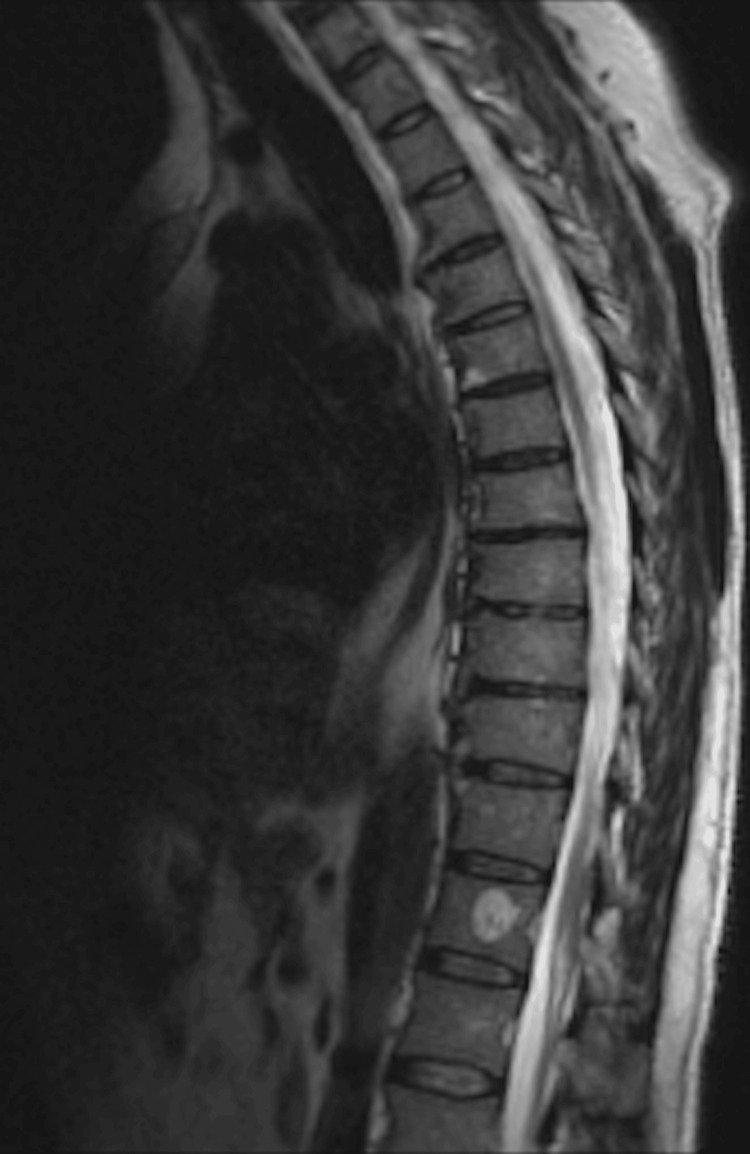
MRI of the thoracic and lumbar spine Sagittal MRI demonstrating degenerative changes without evidence of osseous or epidural metastatic disease.
